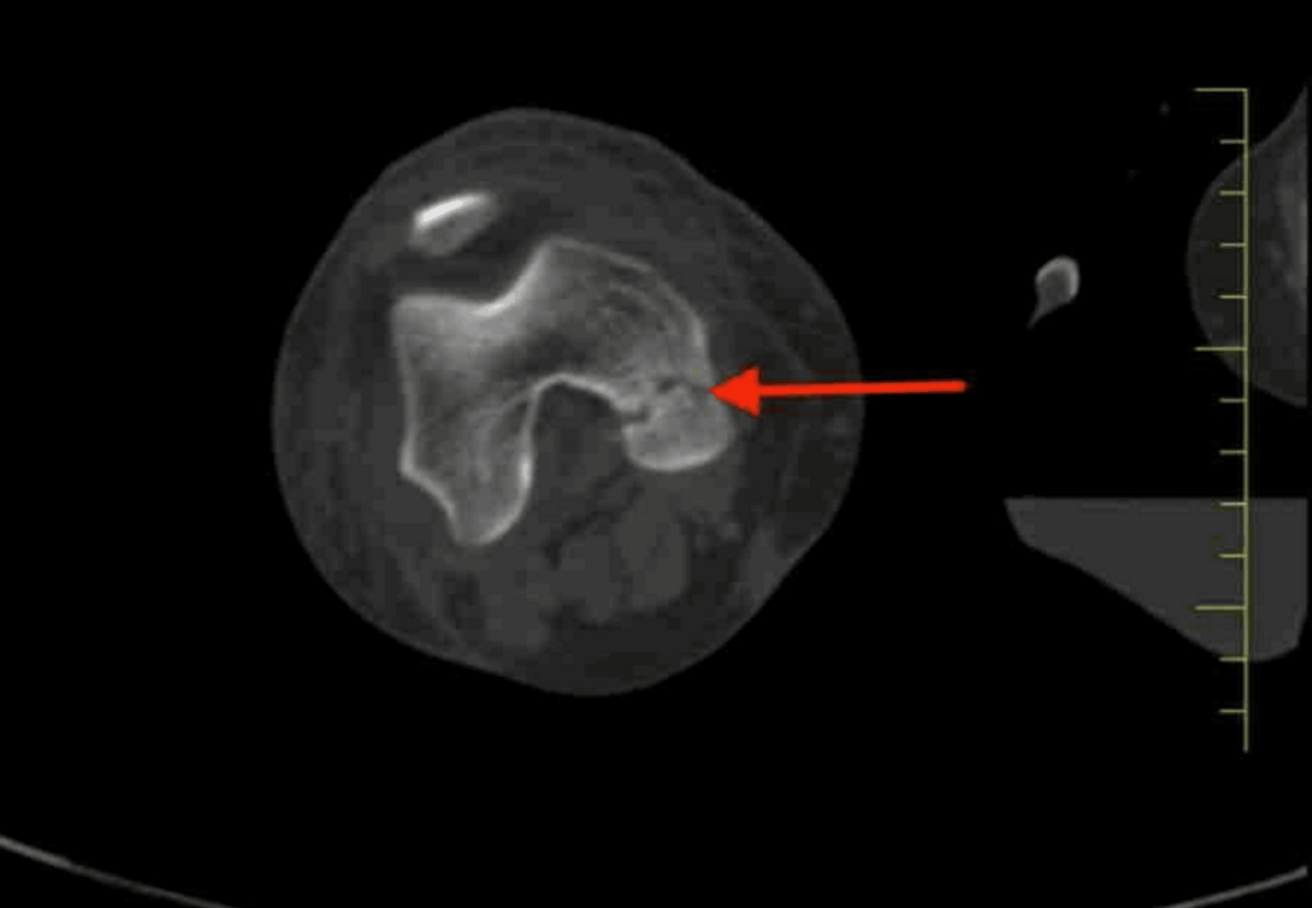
MRI scan showing avulsion fractures of the ACL and PCL at their tibial attachments ACL, anterior cruciate ligament; PCL, posterior cruciate ligament
